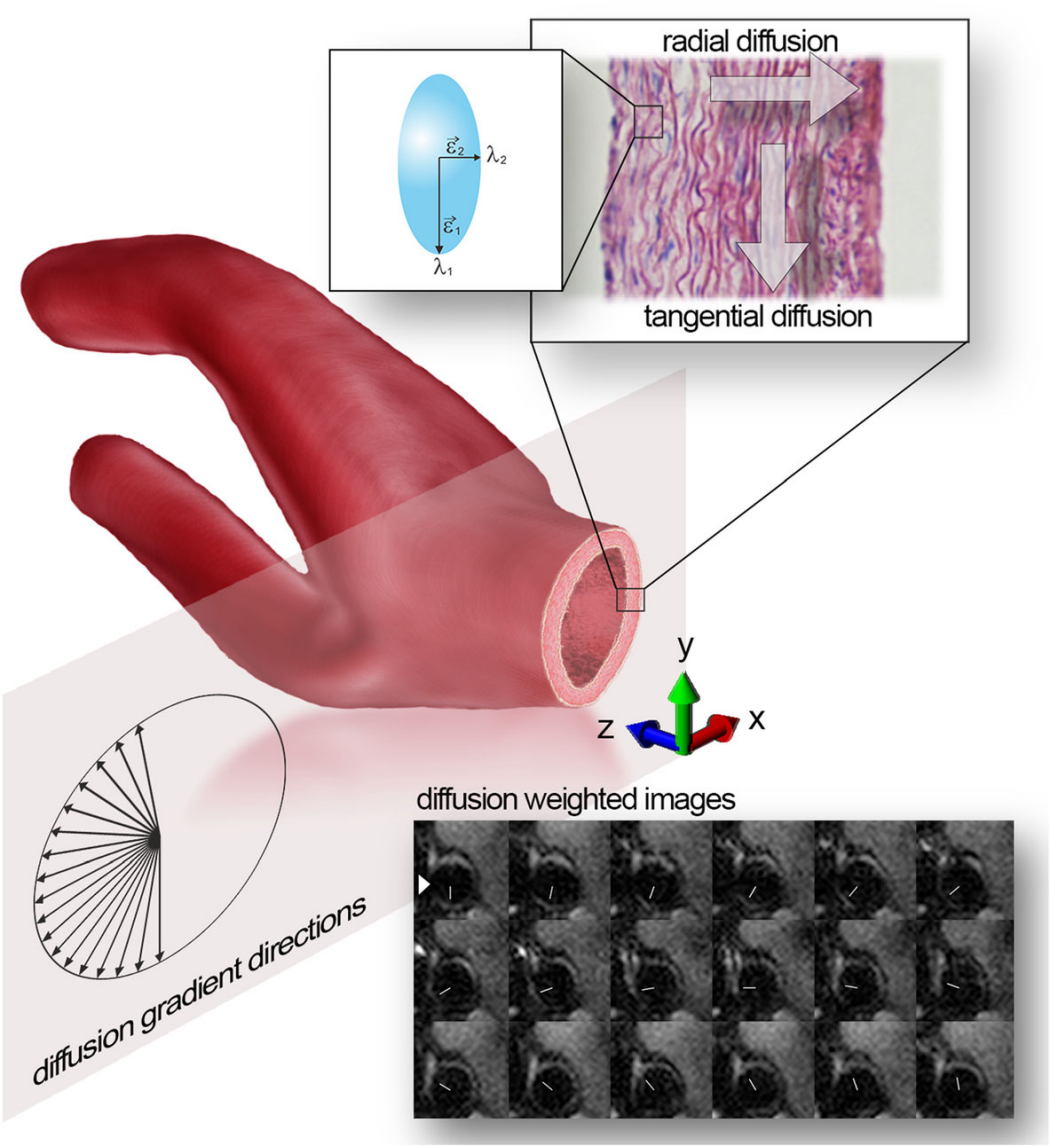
Gradient direction scheme to measure tangential and radial diffusion. *Top*, The concept of tangential and radial diffusion is outlined as well as the 2D ellipsoid with eigenvalues (λ) and eigenvectors (ε). *Middle*, The sketch outlines the anatomical region of interest (carotid artery) and the orientation of the 18 diffusion gradient directions on a hemicycle within a plane perpendicular to the longitudinal axis of the carotid artery (*black arrows*). *Bottom*, Representative in vivo DWI from a carotid artery at a b-value of 600 s/mm^2^ for the 18 diffusion directions (*white headless arrows*)
